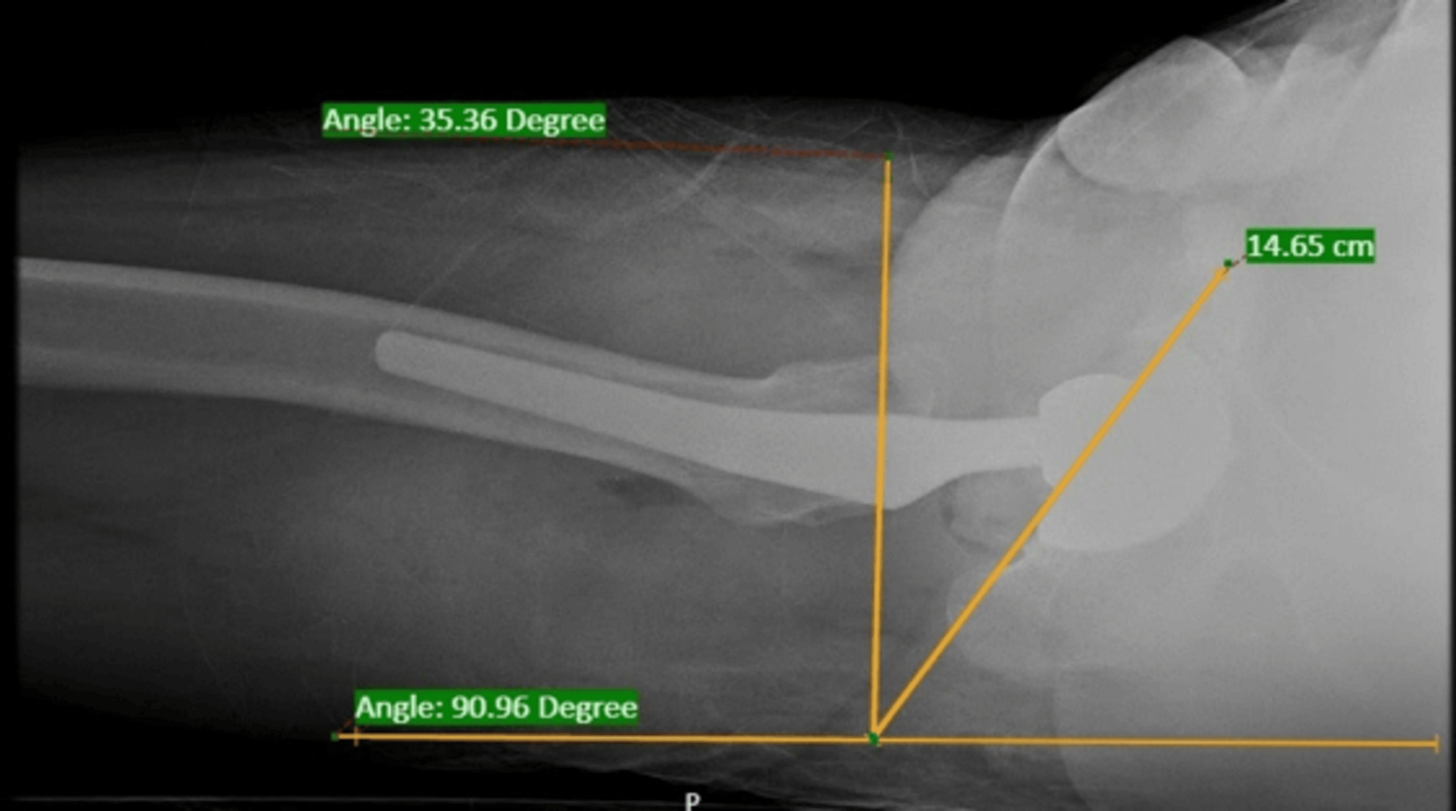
Cross-table radiograph done in our study
